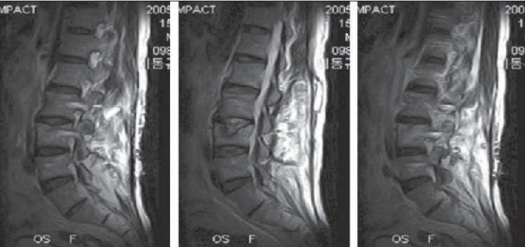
L_3_ burst fracture: MRI taken at postoperative 13 months, demonstrates the nondegenerated L_3-4_ disc with pseudo-Schmorl's node in the upper end-plate of L_4_
